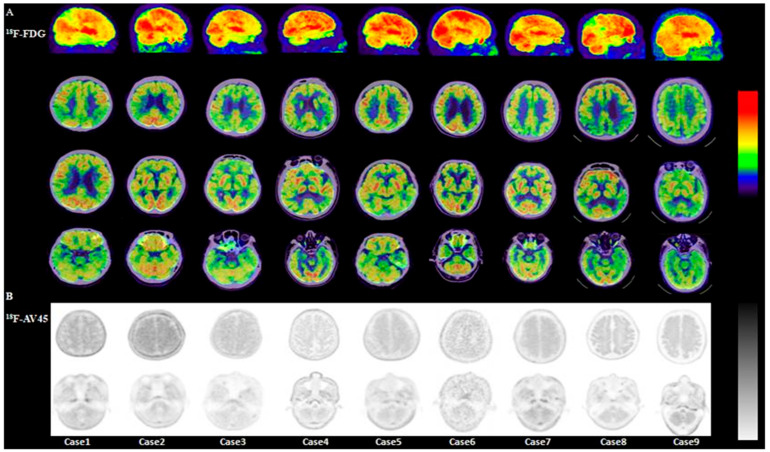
^18^F-AV45 PET imaging in 12 patients with atypical ^18^F-FDG PET/CT findings or DLB symptoms showed diffuse cortical Aβ deposition in 9. ^18^F-FDG PET/CT findings in these patients were: Case 1: posterior cingulate hypometabolism, medial temporal hypometabolism; Cases 2–7: medial temporal hypometabolism; Case 8: medial temporal and parietal hypometabolism; Case 9: global hypometabolism.
